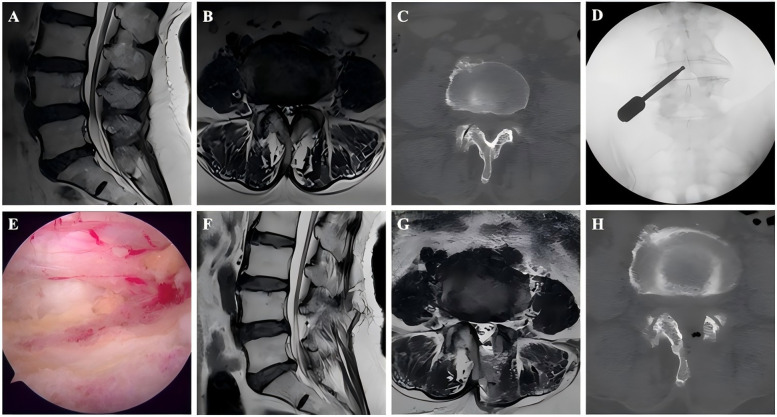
Images from a non-ASD patient treated with UBE. A–B: Preoperative MRI showed L4–L5 LSS. C: Preoperative CT. D: The position of the grinding drill was shown in the fluoroscopic view. E: The nerve was completely decompressed. F–G: Postoperative MRI confirmed spinal canal decompression. H: Postoperative CT showed a lamina defect after partial laminotomy.
